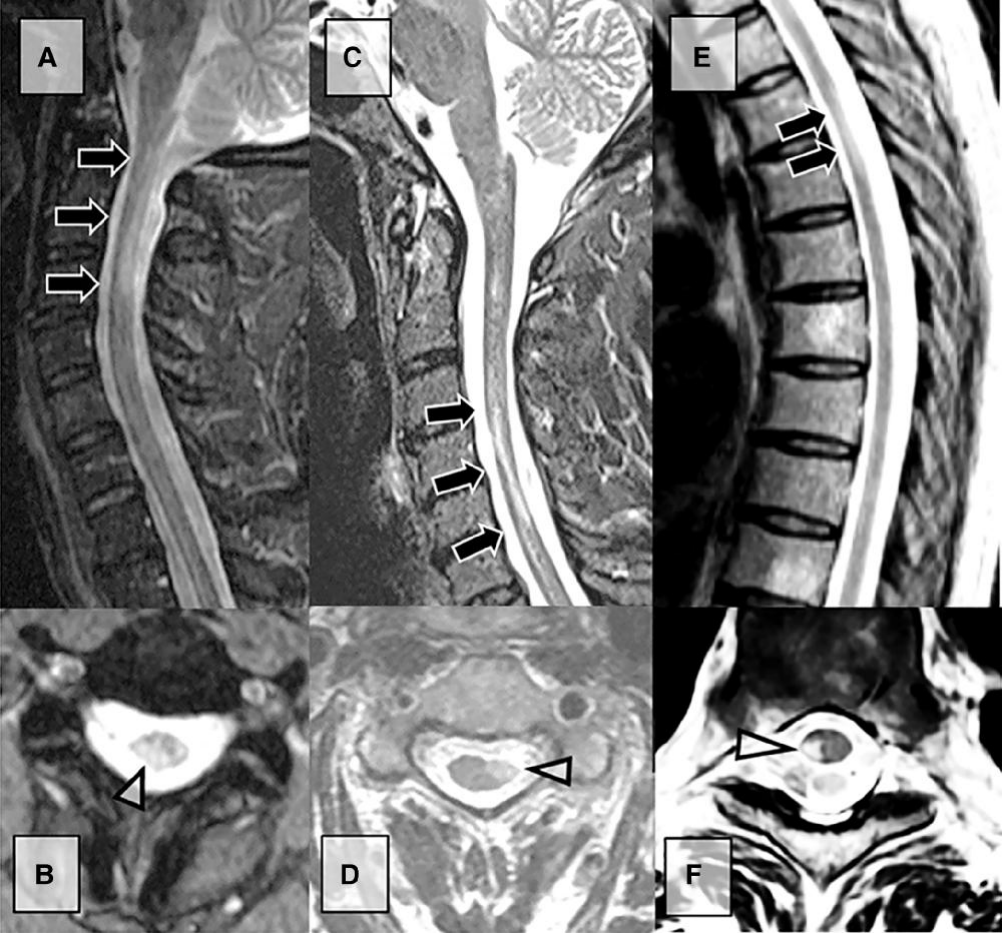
Critical demyelinating lesions of the spinal cord. ‘Critical’ demyelinating lesions of the spinal cord are anatomically associated with progressive motor impairment in people with multiple sclerosis; they are typically laterally based in the corticospinal tract and have focal spinal cord atrophy. MRI cervical spine sagittal (A) and axial (B) images showing ‘critical’ demyelinating lesion with T2 signal abnormality in the right lateral-dorsal cord with focal atrophy (black arrows and arrowhead) in a 53-year-old man with a 5-year history of progressive right upper motor neuron face-sparing hemiparesis. CSF showed four unique oligoclonal bands. MRI cervical spine sagittal (C) and axial (D) images showing ‘critical’ demyelinating lesion with T2 signal abnormality in the left lateral cord (arrows) with focal atrophy (open arrowhead) in a 59-year-old woman with a prior history of relapsing-remitting multiple sclerosis and an 8-year history of progressive left face-sparing upper motor neuron hemiparesis. Additional demyelinating lesion was seen in the lower thoracic spine with no volume loss (not shown). MRI thoracic spine sagittal (E) and axial (F) images showing ‘critical’ demyelinating lesion with T2 signal abnormality right hemispinal cord (arrows and open arrowhead) in a 58-year-old man with multiple sclerosis and progressive right lower extremity monoparesis.
